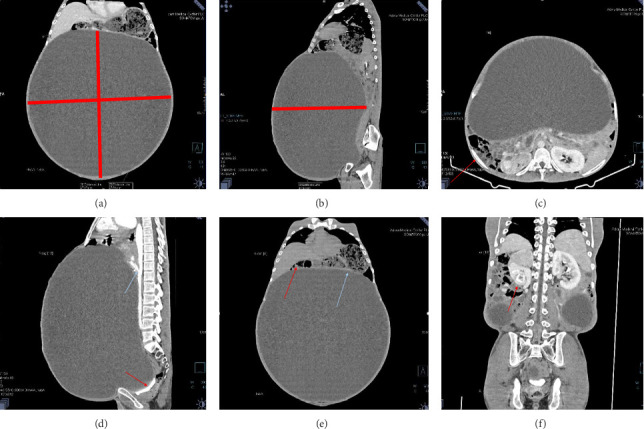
Contrast-enhanced CT of the abdomen showing a well-defined, large cystic lesion measuring 43.1 × 43.0 × 31.26 cm. (a, b) Axial and sagittal views of the cyst, (c) compression and displacement of intestinal loops, (d) compression of major vessels (blue arrow) and urinary bladder (red arrow), (e) displacement of the liver (red arrow) and spleen (blue arrow), (f) right-sided severe hydronephrosis.
